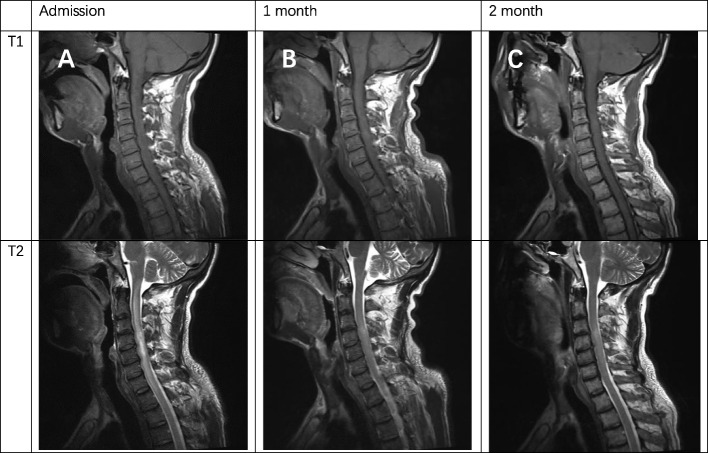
MRI of spinal cord abscess changes during treatment. A-C (from onset to 2 months) showed progressive reduction of cerebral lesions with prolonged treatment
